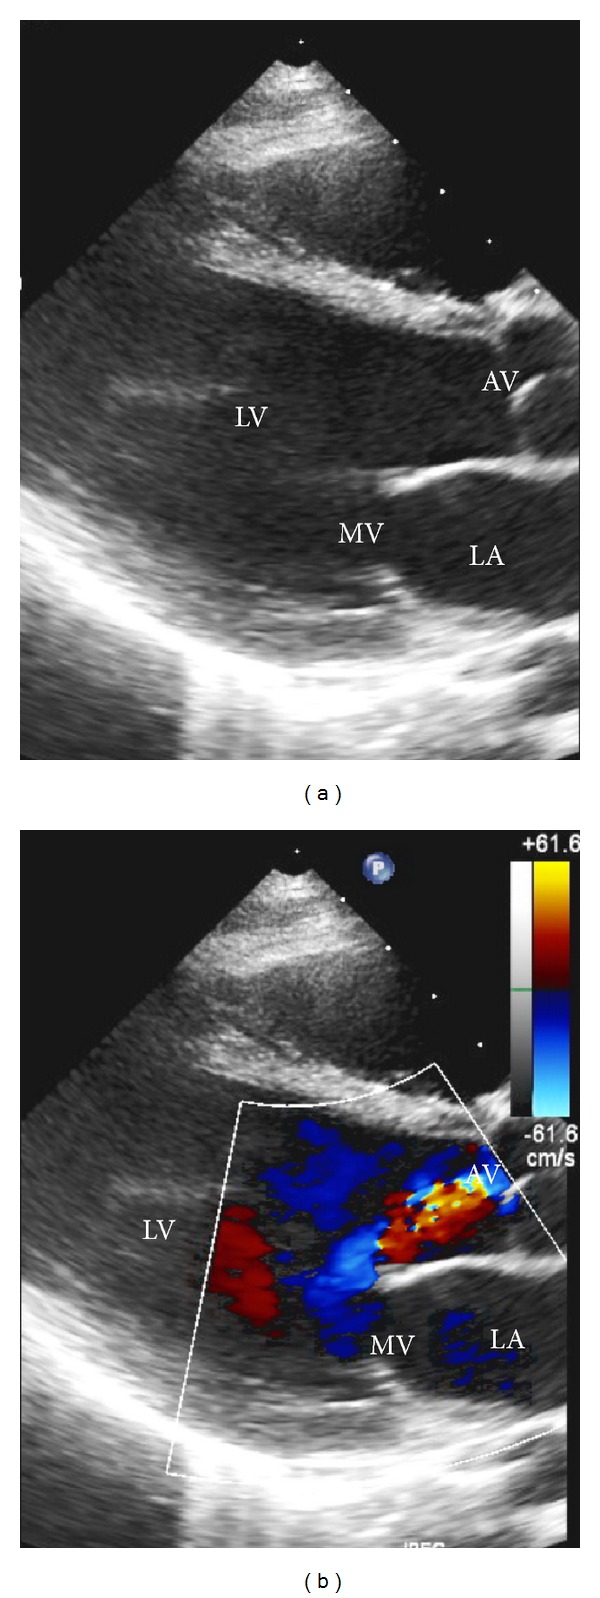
Parasternal long axis view showing (a) orientation of the aortic valve (AV), left ventricle (LV), and left atrium (LA) and (b) aortic valve (AV) and mitral valve (MV) regurgitations demonstrated by blue jets of retrograde flow on Doppler.
